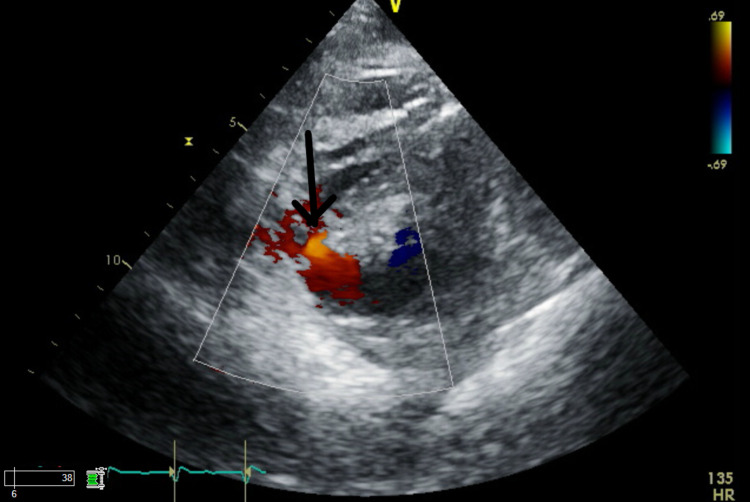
Color Doppler mode showing flow across ventricular septal defect
